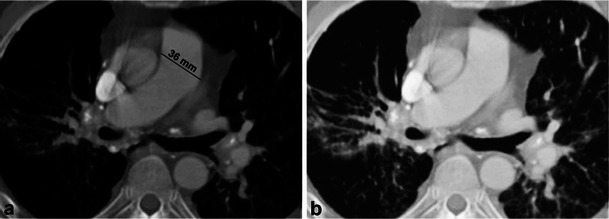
Axial CT images in a patient with prior histoplasmosis demonstrates an enlarged pulmonary trunk (36 mm). The proximal right and left pulmonary arteries are normal in calibre but taper and are severely narrowed at the level of hila. In addition, there are calcified mediastinal lymph nodes, calcified pulmonary granulomas and interlobular interstitial thickening. These findings represent fibrosing mediastinitis
